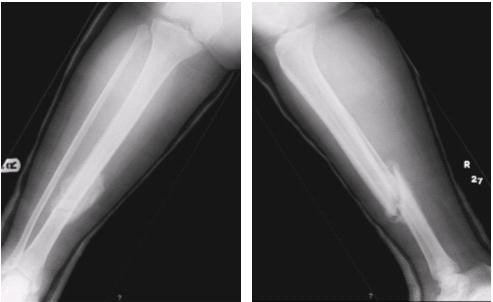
Radiograph following caesarean section, 4 weeks post injury.
